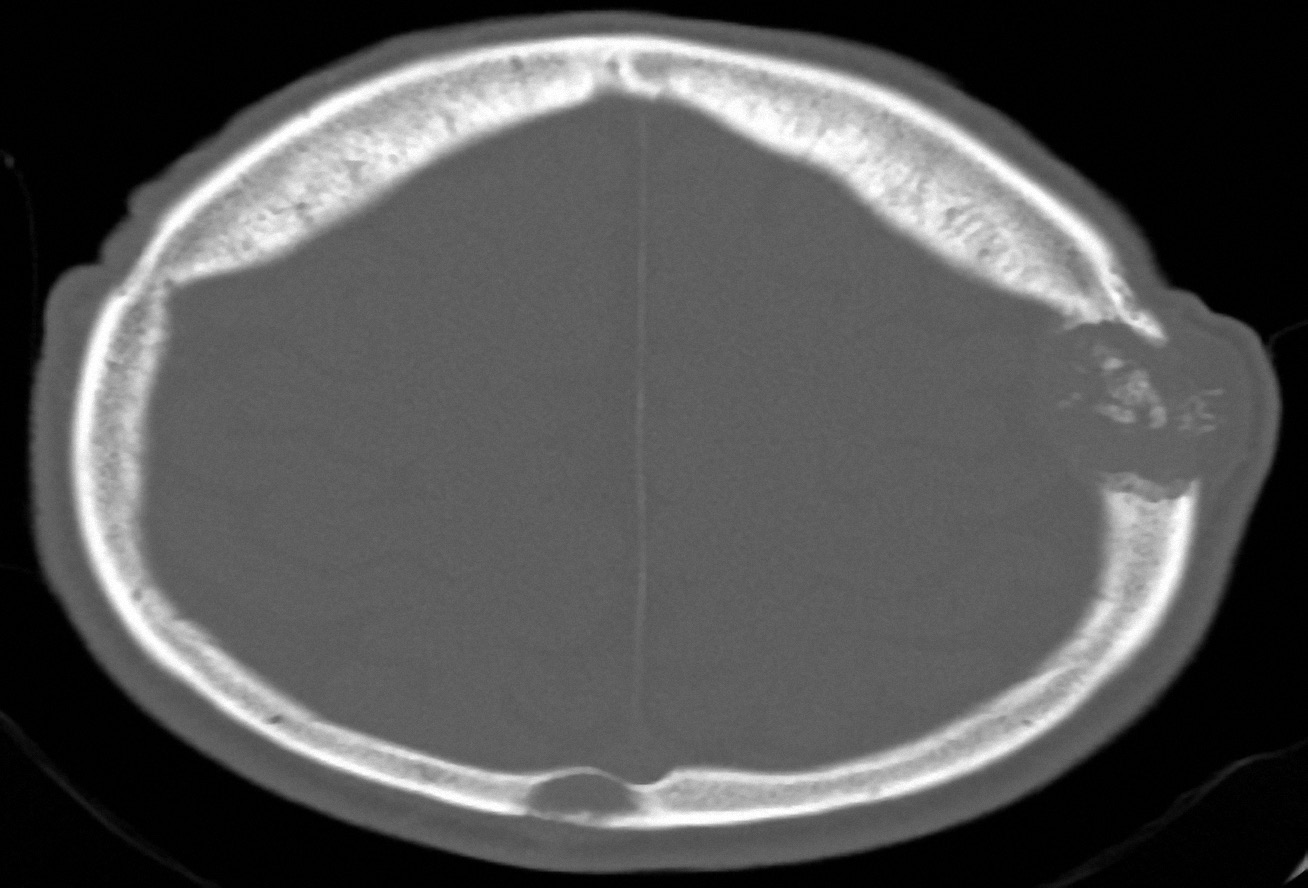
Cerebral CT scan – bone window: osteolytic lesion in the left frontal bone.
